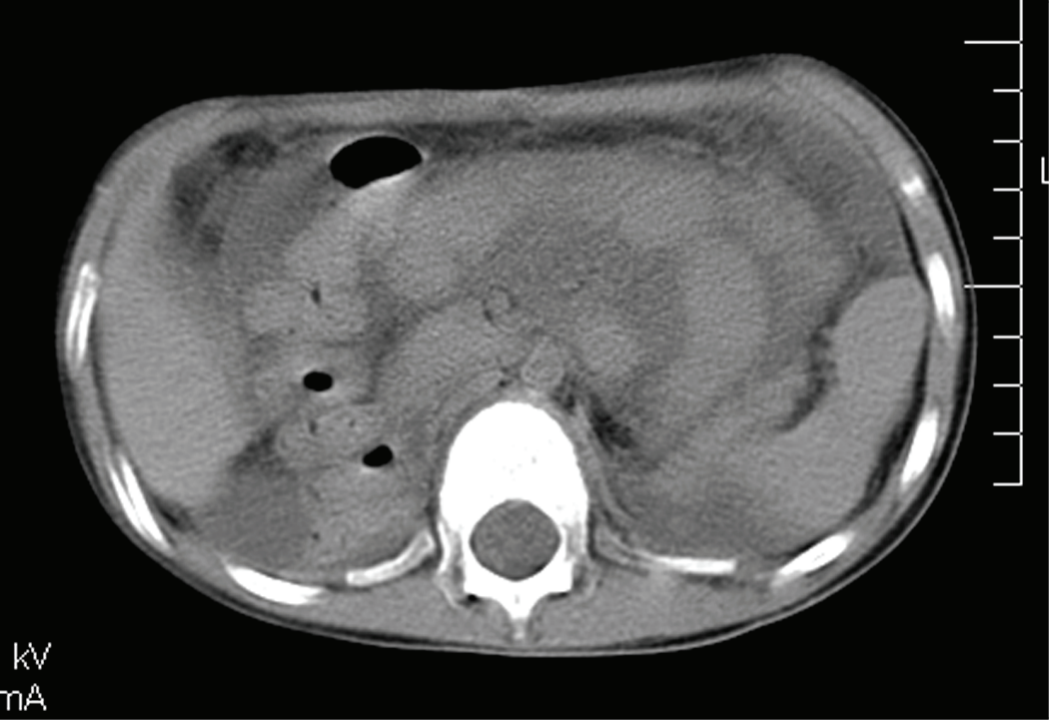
March 2005 - normal cranial, thoracic and abdominal CT scan.
